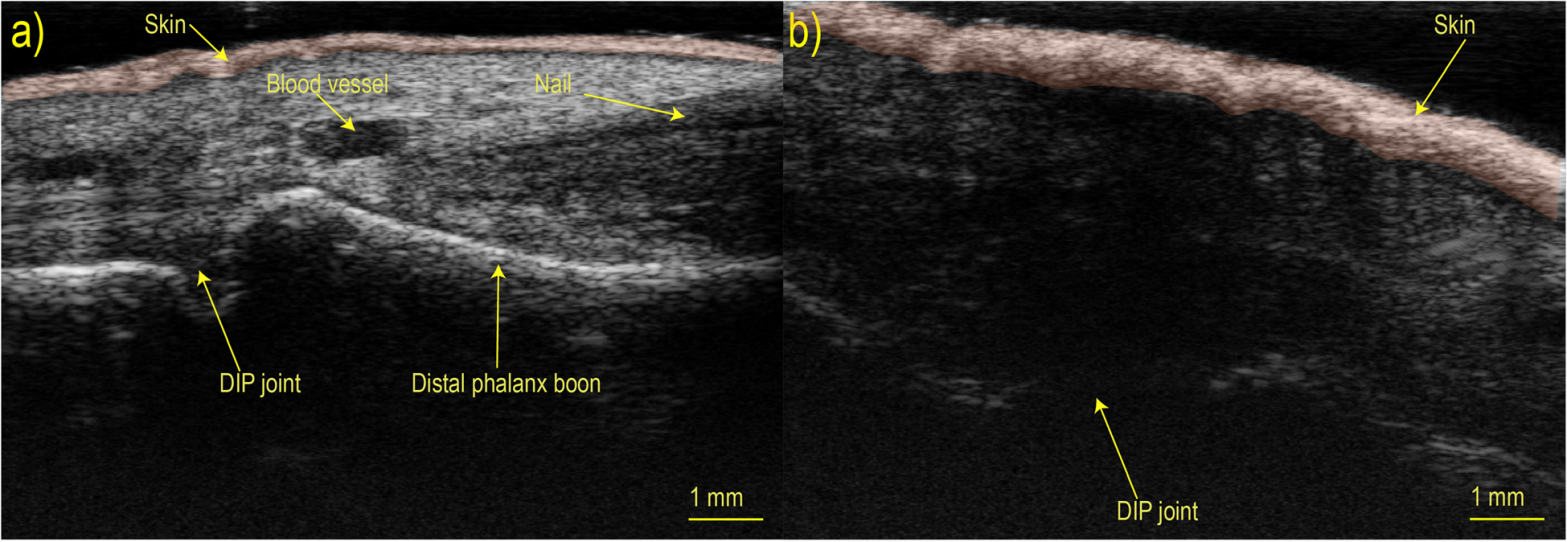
HFUS image of the skin thickening of a healthy volunteer and a SSc patient between the nailfold and the distal interphalangeal joint of the third finger. DIP joint, distal interphalangeal joint. High-frequency ultrasound image of the finger with a notation indicating different parts of the finger. The image a is for healthy volunteer and image b is SSc patient
